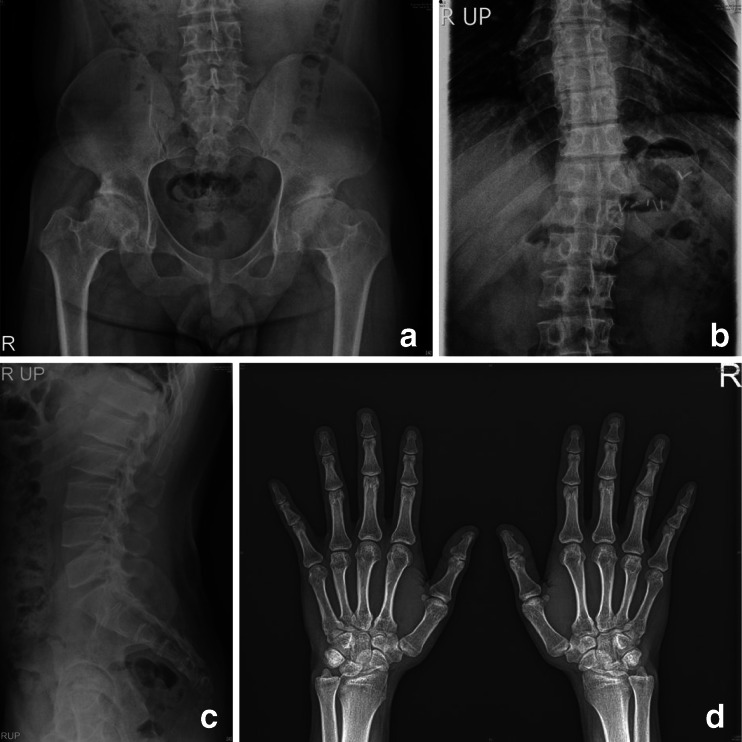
Radiographs of patient 3, male, MPS IVA. a AP pelvis and hips, age 27 years: mildly dysplastic CFE with joint space narrowing; sclerosis (degenerative arthrosis). b AP spine and lower ribs, age 27 years: scoliosis; normal paddle-shaped ribs (T9–T12). c Lateral spine, age 27 years: several superior humped vertebral bodies in the lumbar spine. d AP hands and wrists, age 27 years: normal
